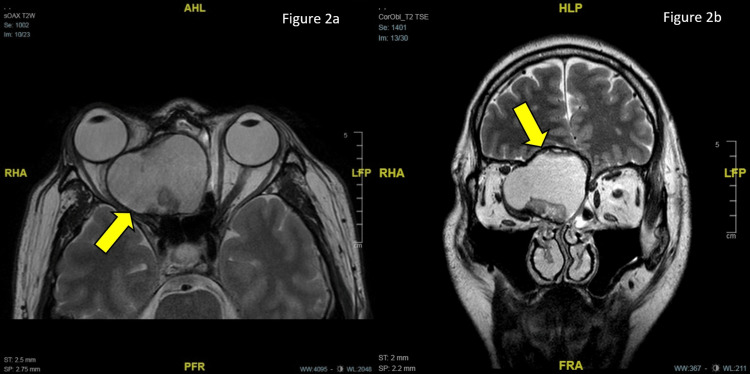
Preoperative axial (a) and coronal (b) T2-weighted MRI image of the brain showing hyperintense lesion in the ethmoidal sinuses with intracranial and right intraorbital extension, right eye proptosis as well as mass effect affecting the right orbital apex.
